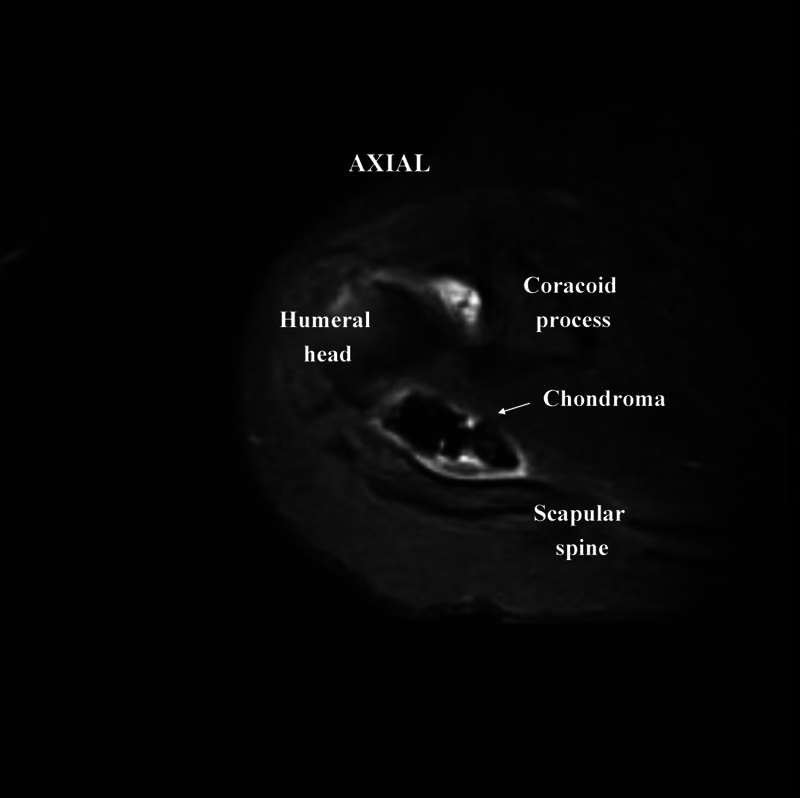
Magnetic resonance imaging of the shoulder (axial view, T2-weighted image)
